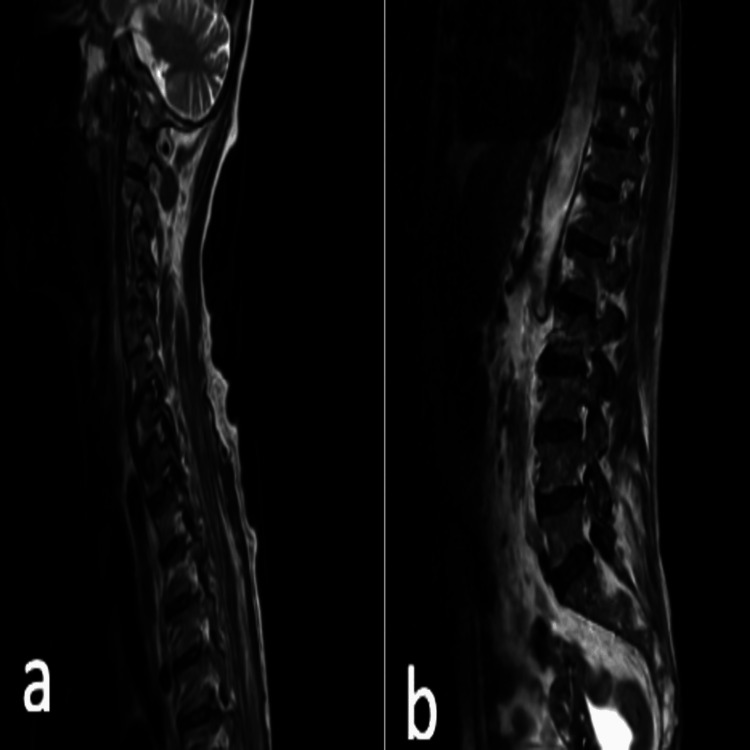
T2 sagittal MRI showing multilevel degenerative spondylotic changes in the cervical and lumbar spine. (a) Cervical MRI and (b) lumbar MRI.
